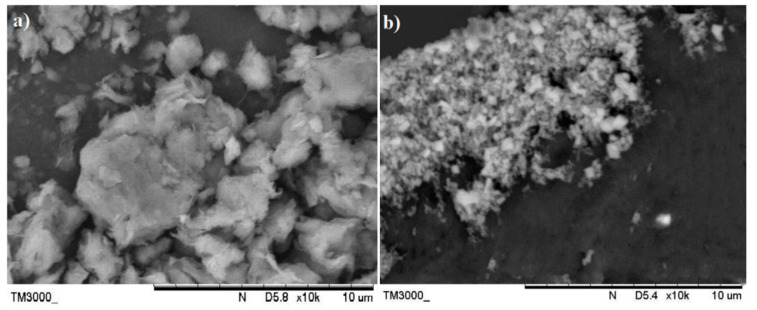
SEM picture of: nano-bentonite (before ultrasonication) (a); nano-bentonite (after ultrasonication) (b), taken at ×10k.
